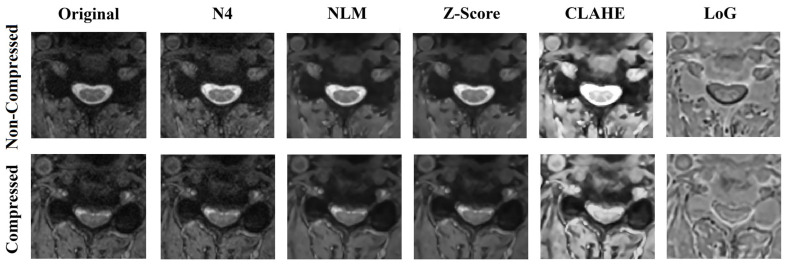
Examples of axial T2*-weighted SC MRI slices showing raw data (left) and the effect of each preprocessing technique.
